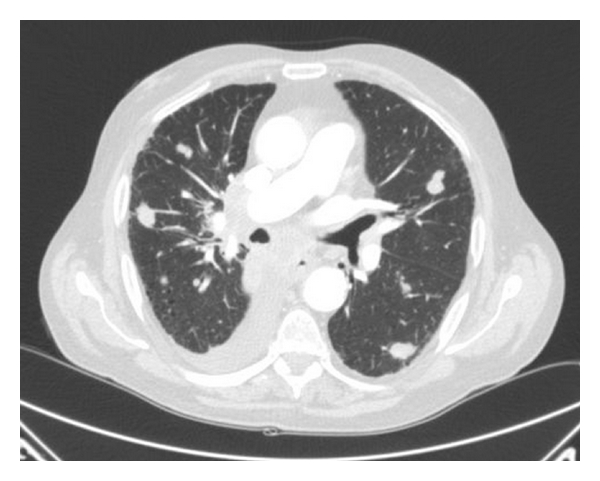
CT scans with multiple bilateral nodules and right pleural effusion.
